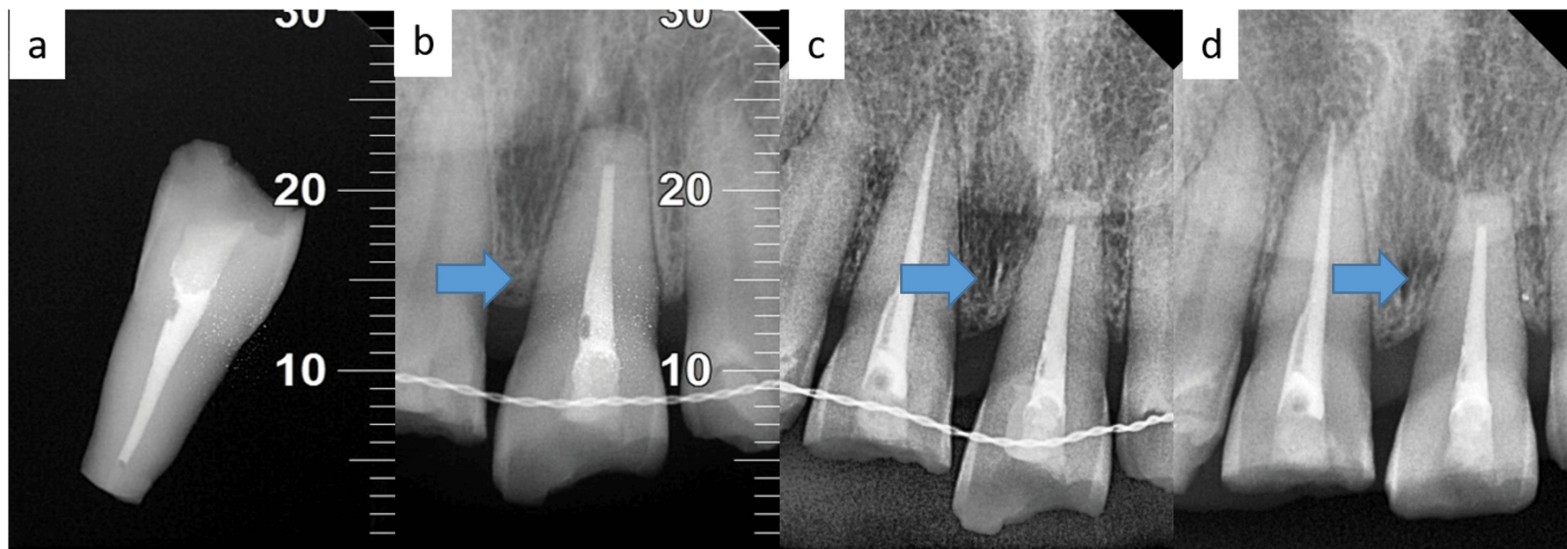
(a) Root canal treatment of tooth 21; (b) Radiograph taken after replantation of tooth, and splinting was done in relation to tooth 21 (blue arrow: avulsed tooth); (c) One-year follow-up (blue arrow: avulsed tooth); (d) Two-year follow-up (blue arrow: avulsed tooth). The markings in Figures 2a-2b serve as anatomical reference points, facilitating the accurate repositioning and verification of the avulsed tooth in relation to the adjacent dentition, thereby ensuring optimal occlusal and spatial relationships.
